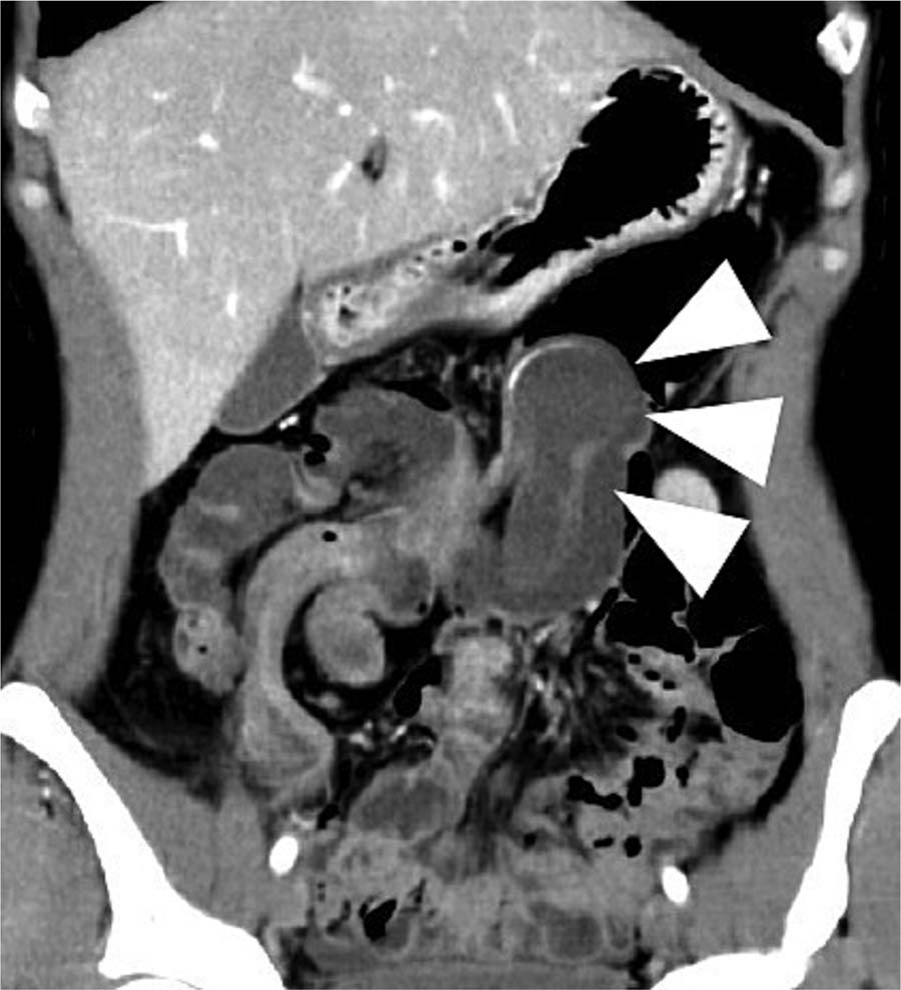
Contrast-enhanced CT demonstrating ileocecal intussusception with a 34-mm cystic lesion at the lead point, indicated by arrows, consistent with an appendiceal mucocele on coronal view.
